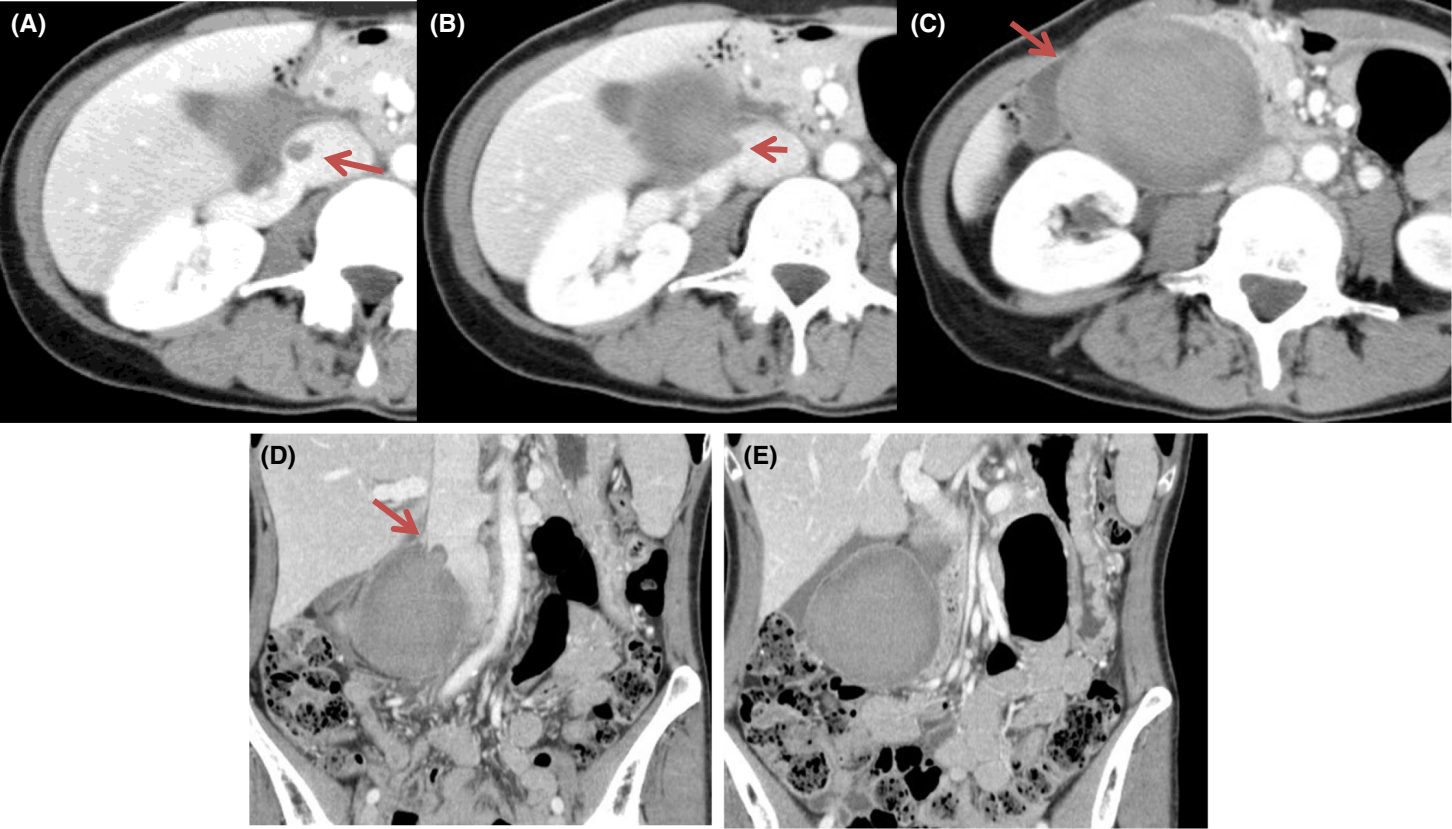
(A–C) Contrast‐enhanced axial CT image demonstrating a 73 × 63 mm homogenous mass lateral to the duodenal second portion and medial to the right kidney with IVC thrombosis. (D, E) Contrast‐enhanced coronal CT image.
